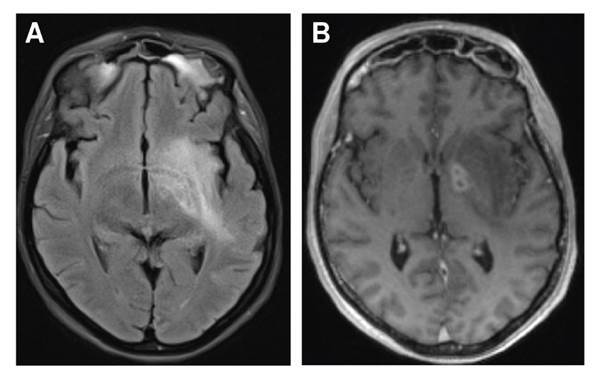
Focos de cerebritis en el ganglio basal en secuencia FLAIR (A) y T1 después del contraste (B)
